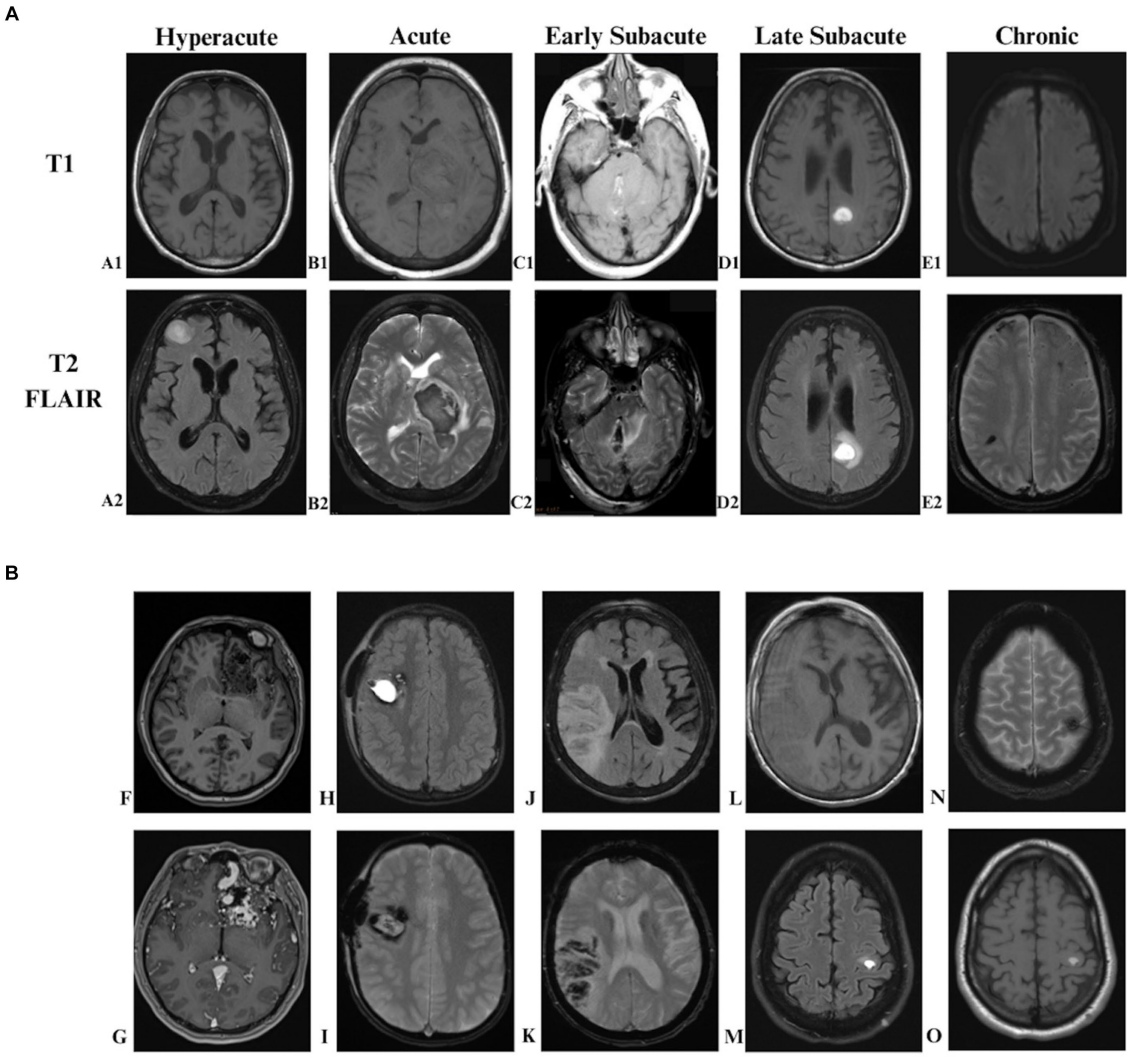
ICH stages of blood over time on MRI and common Etiologies of ICH on MRI. (A) Stages of blood in ICH: (A1) ICH hyperacute blood on T1, (A2) ICH hyperacute blood on T2 FLAIR, (B1) ICH acute blood on T1, (B2) ICH acute blood on T2 FLAIR, (C1) ICH early subacute blood on T1, (C2) ICH early subacute blood on T2 FLAIR, (D1) ICH late subacute blood on T1, (D2) ICH late subacute blood on T2, (E1) ICH chronic blood on T1. (E2) ICH chronic blood on GRE. (B) Common ICH etiologies on MRI: (F) AVM on T1 Pre-Contrast, (G) AVM on T1 Post-Contrast, (H) Cavernous hemangioma on T2 FLAIR, (I) Cavernous hemangioma on GRE, (J) Hemorrhagic conversion of ischemic stroke on T2 FLAIR, (K) Hemorrhagic conversion of ischemic stroke on GRE, (L) Hemorrhagic conversion of ischemic stroke on T1, (M) Hemorrhagic metastasis on T2 FLAIR, (N) Hemorrhagic metastasis on GRE, (O) Hemorrhagic metastasis on T1. ICH = Intracerebral hemorrhage, FLAIR = fluid-attenuated inversion recovery, GRE = gradient-echo, AVM = arteriovenous malformation.
